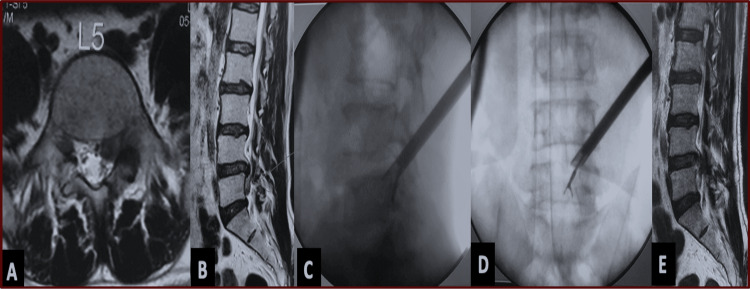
(A, B) Preoperative MRI of a 43-year-old male showing a very high-migrated left-sided disc reaching below the L5 pedicle, Michigan State University type 2AB. (C, D) Intraoperative image intensifier views during surgery performed under local anesthesia via the outside-in technique with endoscopic burred foraminoplasty. Note the highly oblique endoscopic trajectory on both anteroposterior and sagittal planes. (E) Postoperative sagittal MRI T2 image confirming fragment removal and decompression.
